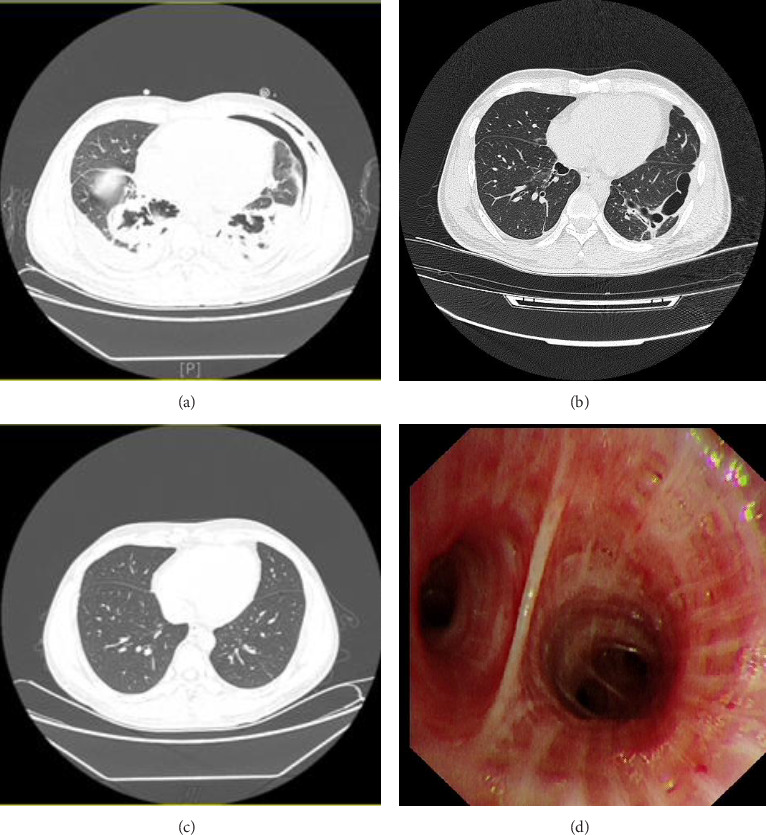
(a) July 14, 2022 chest CT showed a left-sided liquid pneumothorax, right-sided pleural effusion, bilateral lower lobe of lungs with exudation and cavity formation, bilateral pleural thickening and adhesions, and bilateral subcutaneous pneumatosis of the chest wall. (b) August 22, 2022 chest CT showed bilateral lower lobe exudates with cavity formation, left pneumothorax, bilateral pleural effusions, and subcutaneous pneumatosis of the left chest wall, which were less than before. (c) June 12, 2024 chest CT showed significantly better inflammatory lesions in the lower lobes of both lungs than before. (d) August 09, 2022 bronchoscopy revealed bilateral bronchial mucosal congestion and edema, predominantly in both lower lobes, and yellowish secretion was seen in the bronchus of the lower lobe of the left lung.
